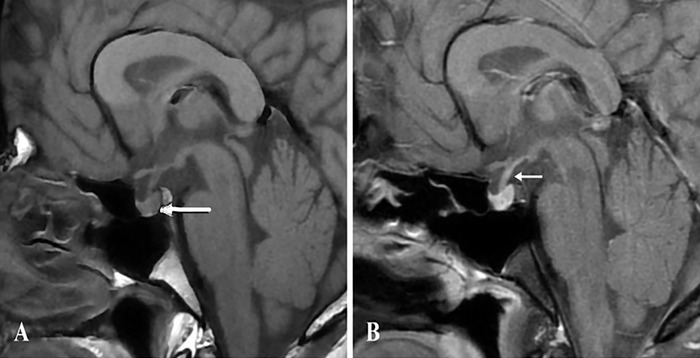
(A) Noncontrast sagittal T1-weighted image shows an absent posterior pituitary bright spot (normal location marked by white arrow). (B) Contrast-enhanced image shows normally enhancing pituitary stalk (white arrow) without any thickening.
